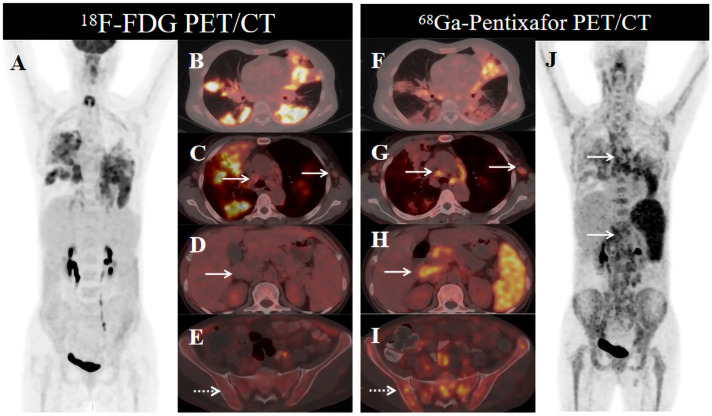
^18^F-FDG and ^68^Ga-Pentixafor PET/CT images of a 60-year-old female with CLL/SLL. ^18^F-FDG PET/CT maximum intensity projection (MIP, A) image shows bilateral patchy pulmonary hypermetabolism (B); no significant hypermetabolism in enlarged mediastinal (C, white arrows), retroperitoneal (D, white arrows) lymph nodes or systemic bone marrow (E, dotted arrow). ^68^Ga-Pentixafor PET/CT MIP (J) image shows mild pulmonary uptake (F), with markedly increased uptake in the above-mentioned lymph nodes (G, H, white arrows) and systemic bone marrow (I, dotted arrow).
